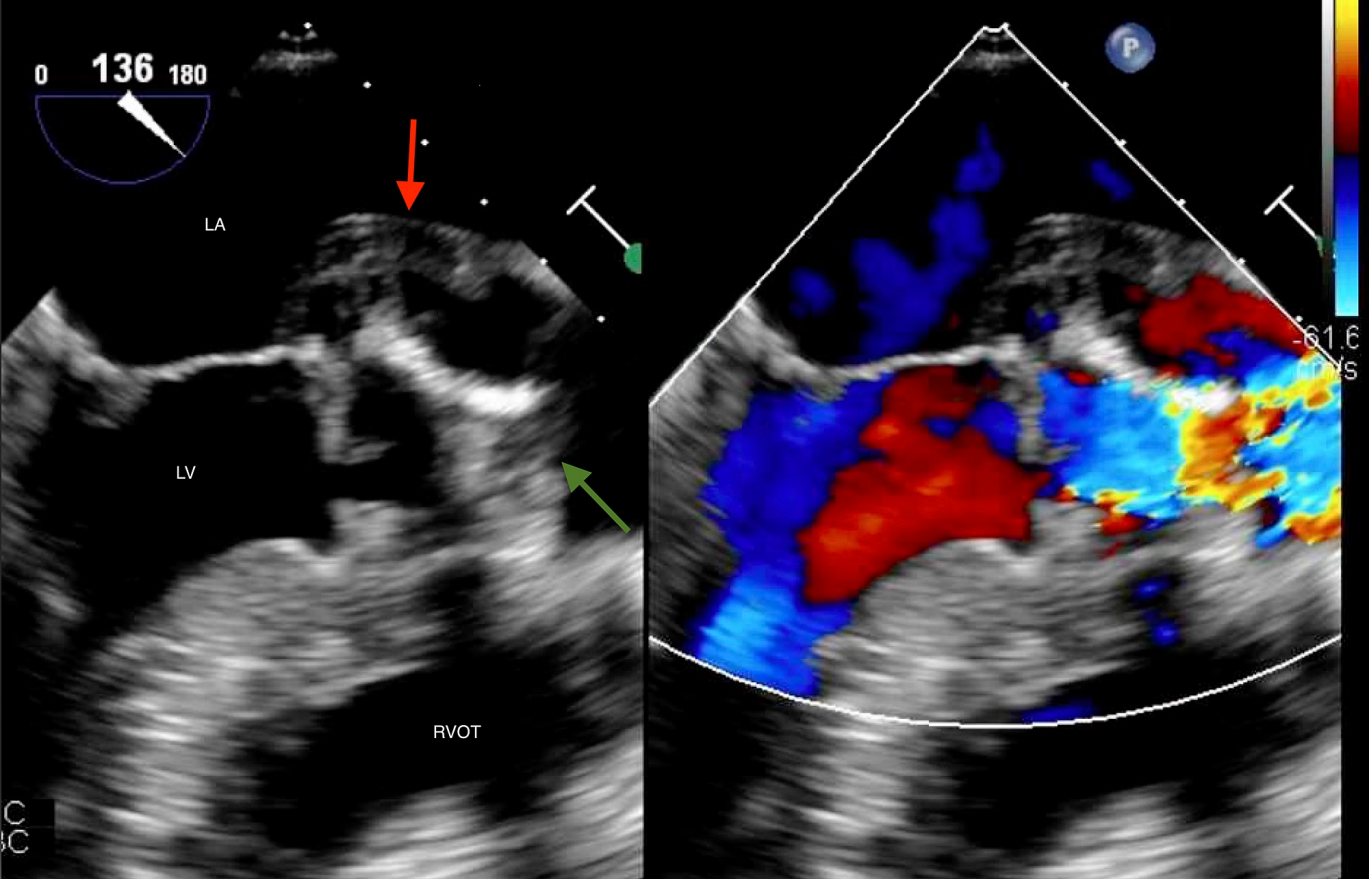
Midesophageal long axis view on TEE shows (green arrow) a vegetation on the aortic valve which leads to significant aortic regurgitation (red arrow). The lucency is highly suspicious for aortic valve abscess formation. TEE, transesophageal echocardiogram
